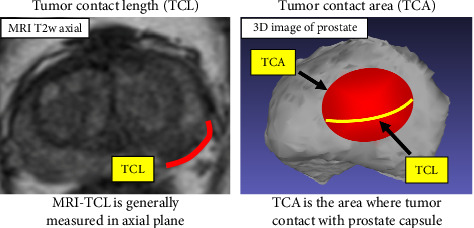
TCL and TCA. TCL is defined as the maximum length of the contour of index lesion which contact with the prostatic capsule. In our study, MRI-TCL was measured in axial plane. TCA is the area where tumor contact with prostate capsule.
